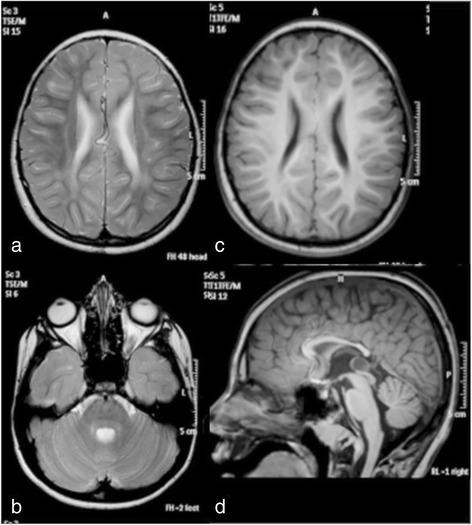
Axial T2 sequences showed slightly increased T2 signal in supratentorial central white matter (a) while the cerebellar white matter looked normal (b). Axial T1-weighted imaging showed normally signaling supratentorial white matter (c). Sagittal T2-weighted imaging revealed a somewhat thin corpus callosum and a small cyst (1.2 cm) of the corpus pineale (d)
